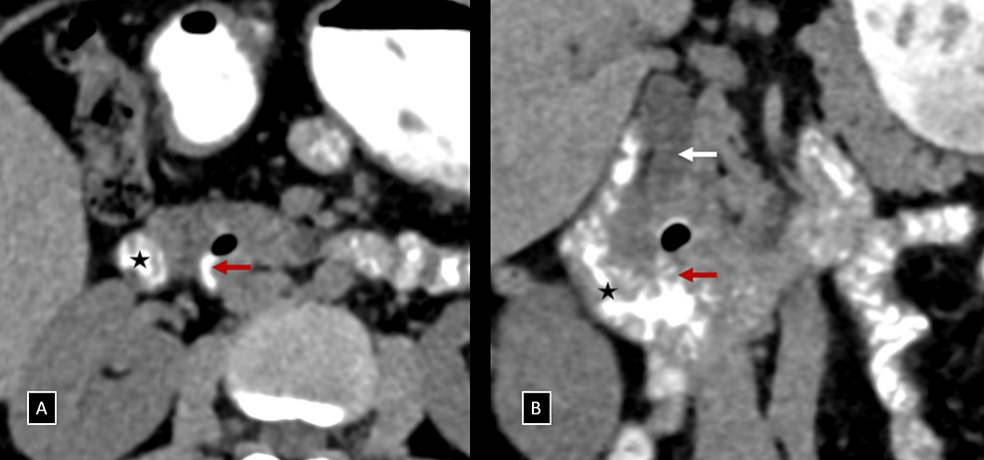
(A and B) Axial and coronal reformatted images of NCCT with positive oral contrast administration demonstrating a small periampullary diverticulum (red arrows) with internal air foci arising from the medial wall of the distal second part/proximal third part of the duodenum (black star), compressing the retro-pancreatic portion of the distal CBD extrinsically (white arrow), just proximal to the ampulla of Vater. NCCT, non-contrast enhanced computed tomography; CBD, common bile duct
